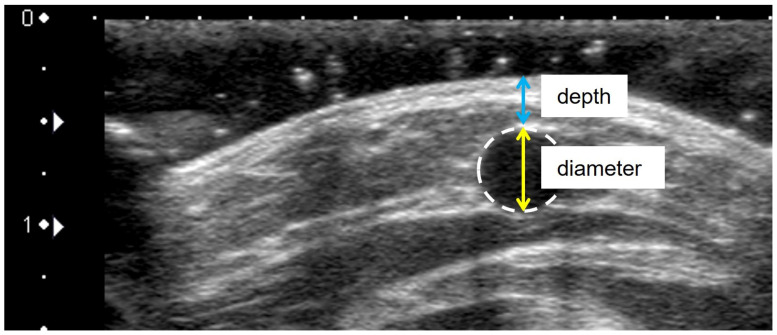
Measurement of venous diameter and depth by ultrasonography. Notes: Venous diameter (mm) was measured from the upper end to the lower end of the vein. Depth (mm) was measured as the vertical distance from the skin surface to the apex of the vein circle.
